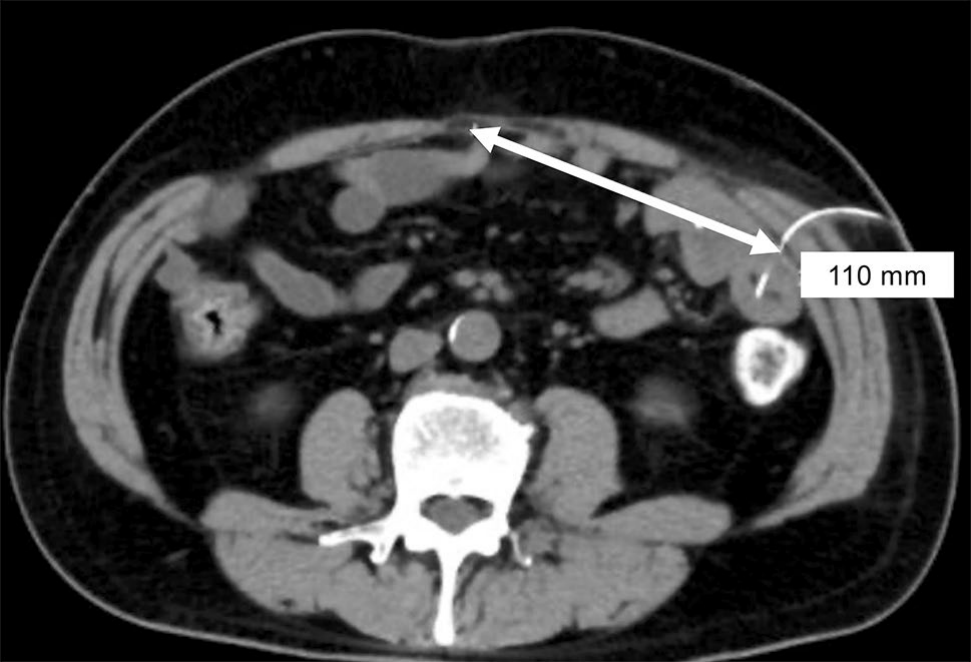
The linear distance between the jejunostomy site and the midline of the abdomen was measured on the CT image.
